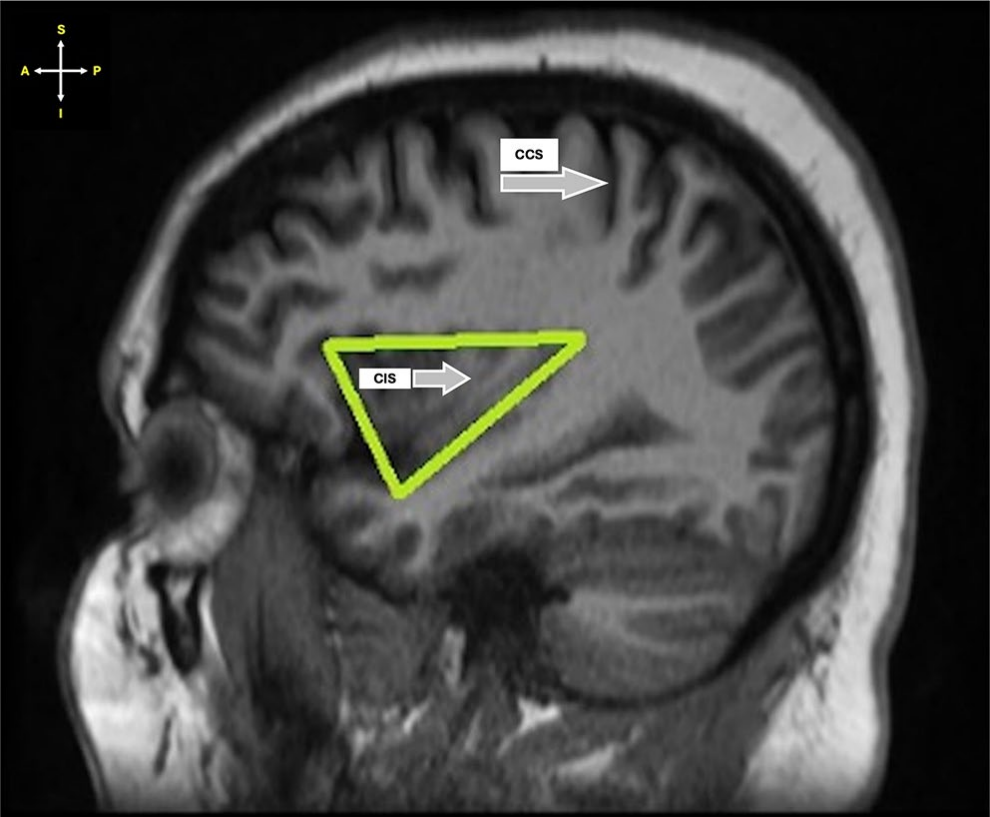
Sagittal section of MRI showing triangular-shaped insula. *Key* CCS – Central cerebral sulcus; CIS – Central insular sulcus
